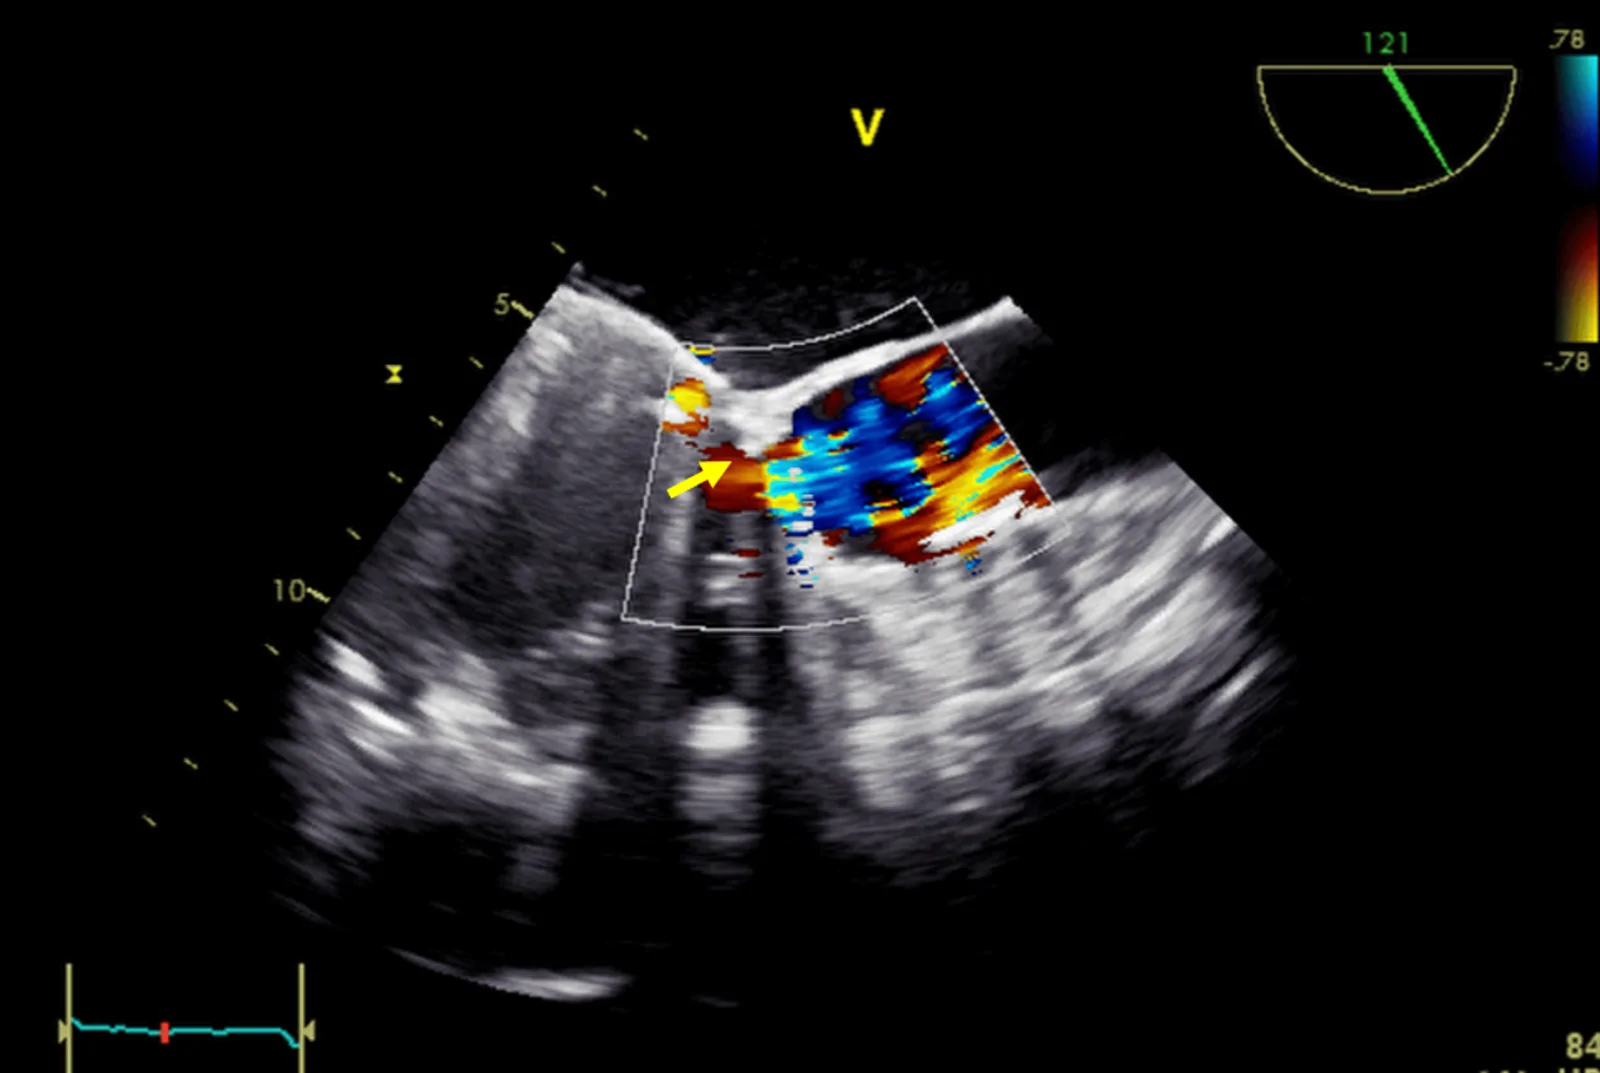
TTE imaging showing a pannus-like mass on the ventricular side of the aortic prosthesis (yellow arrow), associated with marked flow acceleration on color Doppler imaging. TTE, transthoracic echocardiography
